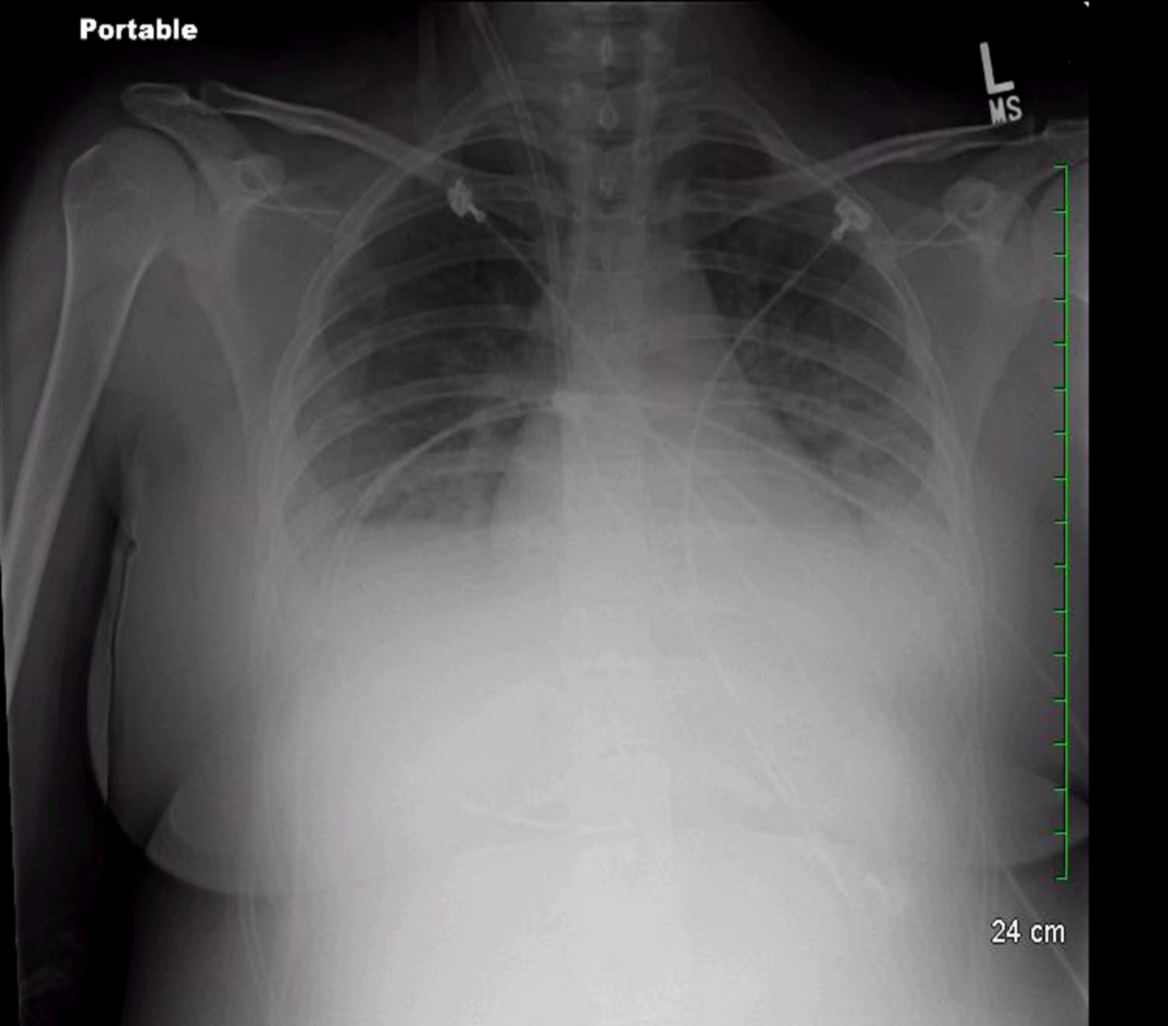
Chest X-ray. Cardiac silhouette is normal in size. Bilateral basilar opacities likely represent moderate pleural effusions.
